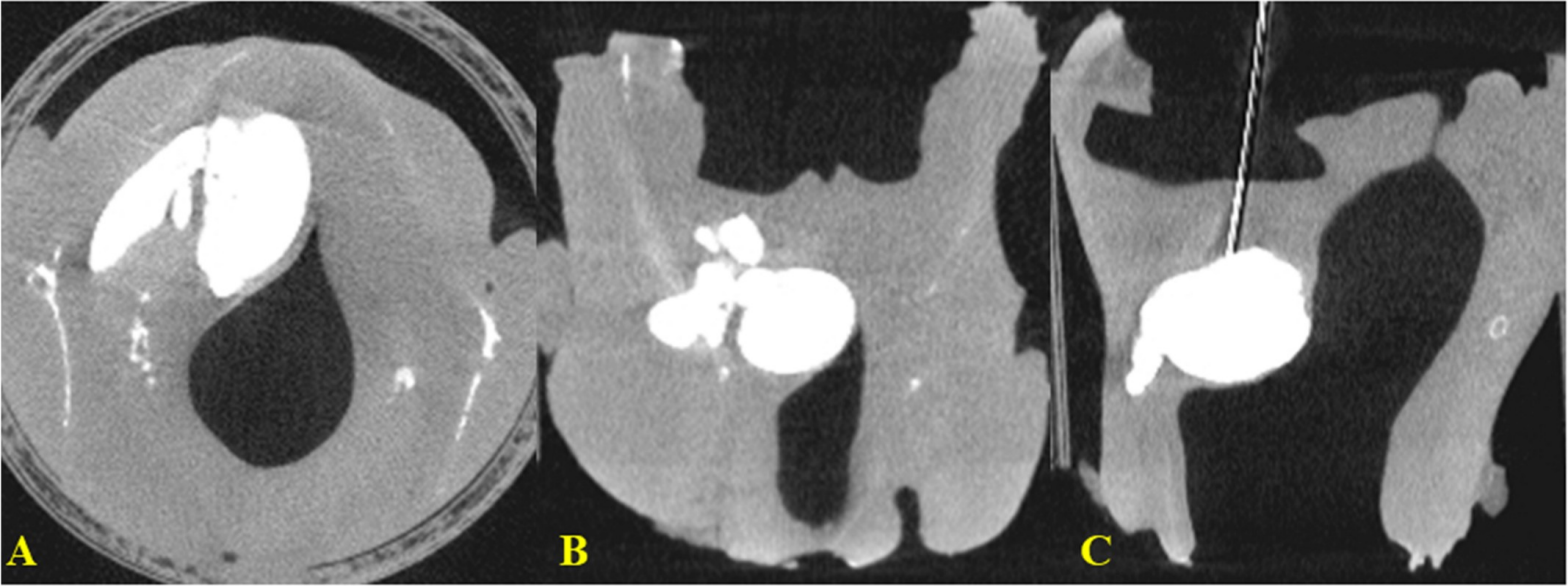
Serial view of micro–computed tomography after paraglottic leak occurred. (A) Axial view. (B) Coronal view. (C) Sagittal view.
